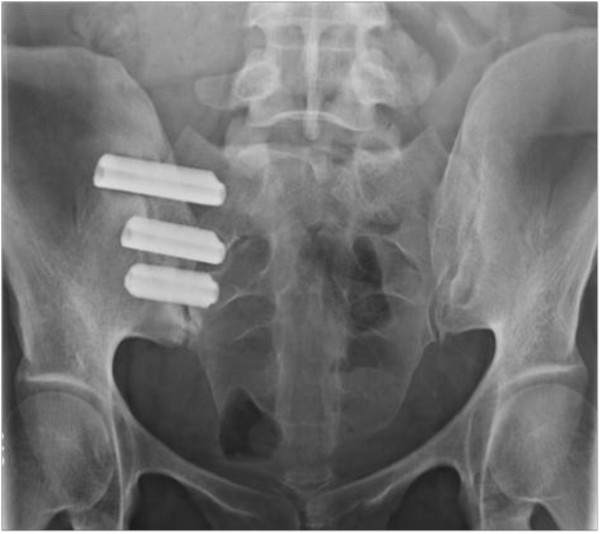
Postoperative radiograph demonstrating placement of 3 implants.
